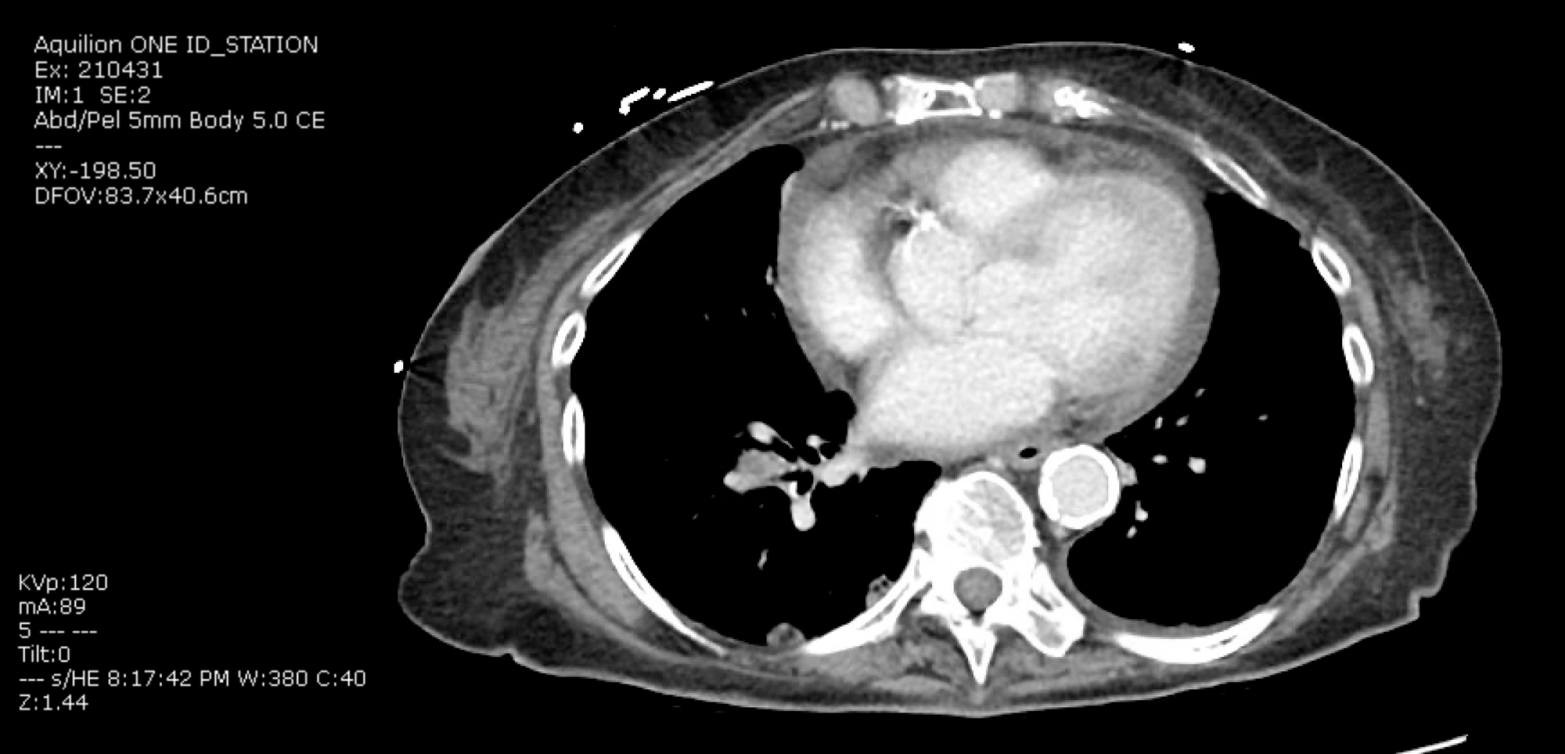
CT Thorax showing moderate pericardial effusion
